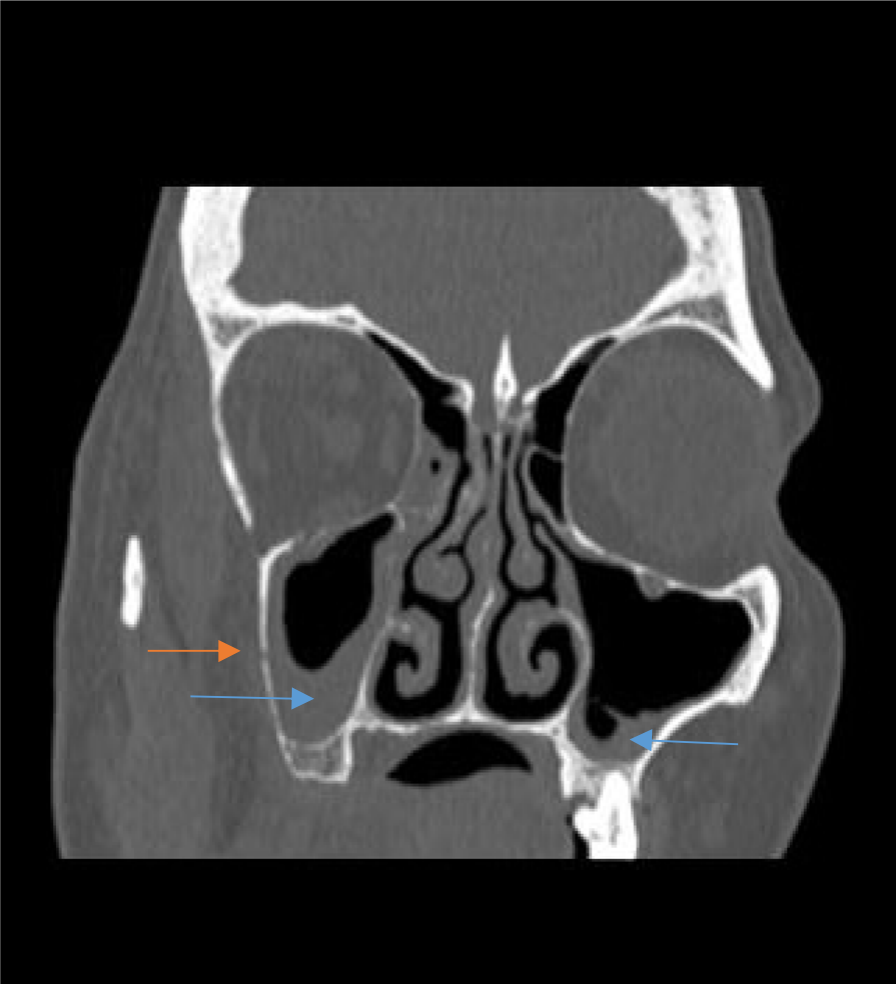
Coronal image of a plain CT of the paranasal sinuses in a patient with sinonasal mucormycosis showing mucosal thickening (blue arrows) in bilateral maxillary sinuses (R > L) with rarefaction and focal erosions (red arrow) in the wall of the right side
